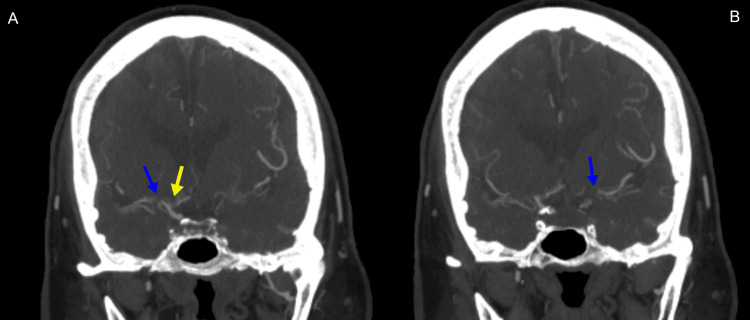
CTA demonstrating bilateral steno-occlusive lesions. CTA demonstrating bilateral steno-occlusive lesions involving the bilateral proximal M1 (blue arrow) and A1 segment (yellow arrow). Panel A demonstrates right-sided pathology, while Panel B demonstrates left-sided pathology. CTA: computed tomography angiography
